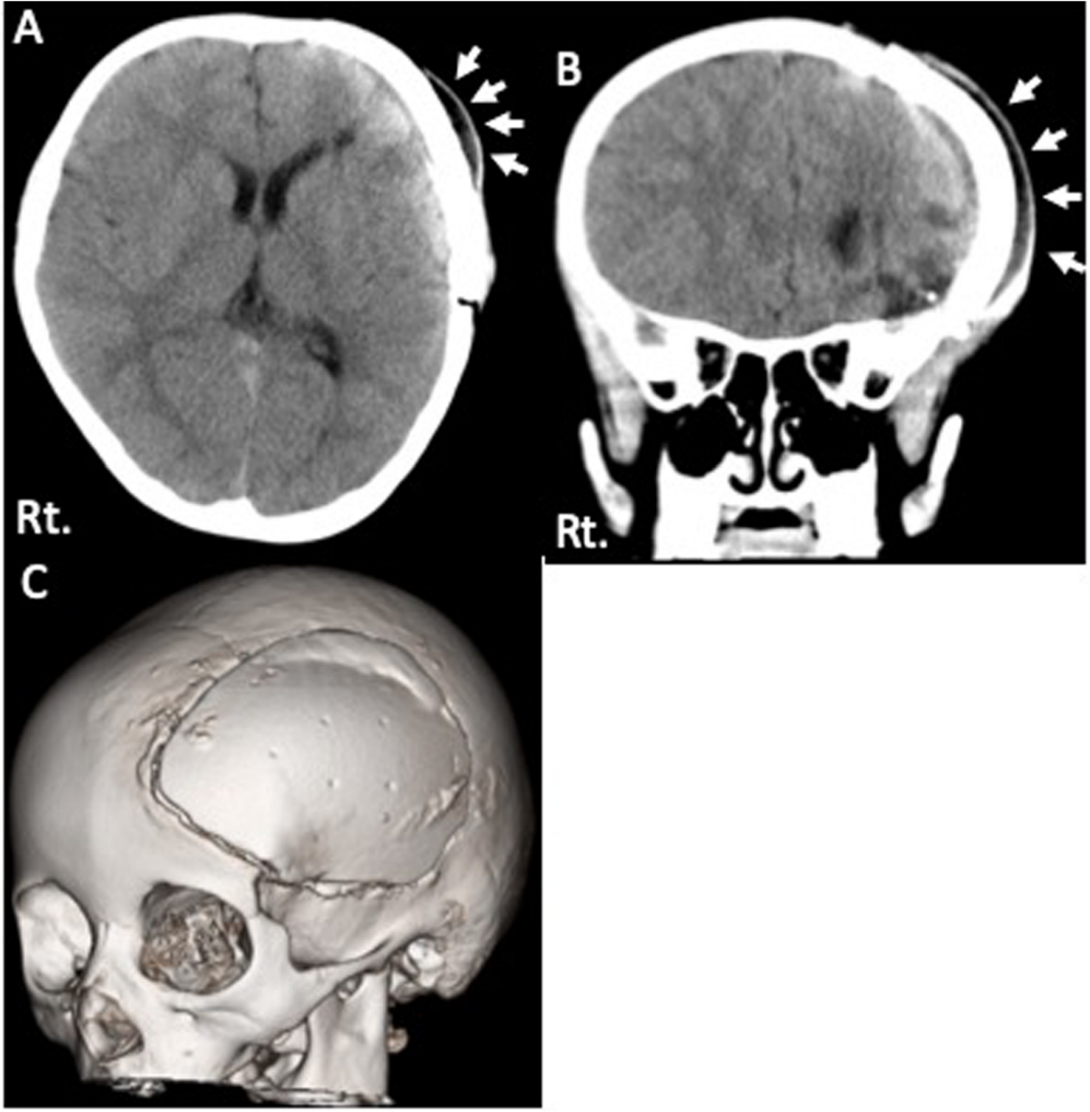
Radiological examination after head injury. a, b Head CT showed subcutaneous fluid collection (white arrows). c Three-dimensional image showed the relationship between the artificial bone flap and skull. No obvious deviation of the bone flap
